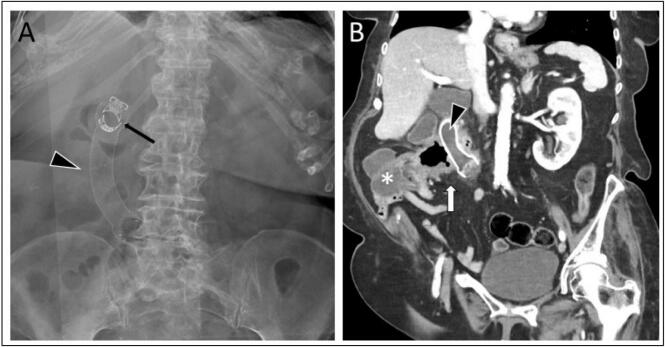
A: Conventional X-ray in anterior-posterior (AP) projection. B: Computed tomography (CT) scan of the abdomen. Diagnostic imaging performed three weeks after the endoscopic placement of a fully covered duodenal stent. The stent is correctly positioned (black arrowhead), and still fixated orally by the two OTSC slips (thin black arrow), hereby covering the opening of the malignant fistula (thick white arrow) created by the ascending colon tumor (*).
